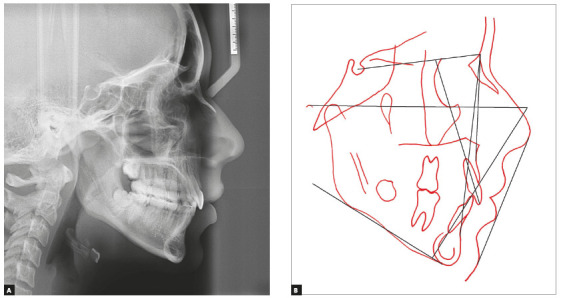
Final cephalometric radiograph (A) and cephalometric tracing
